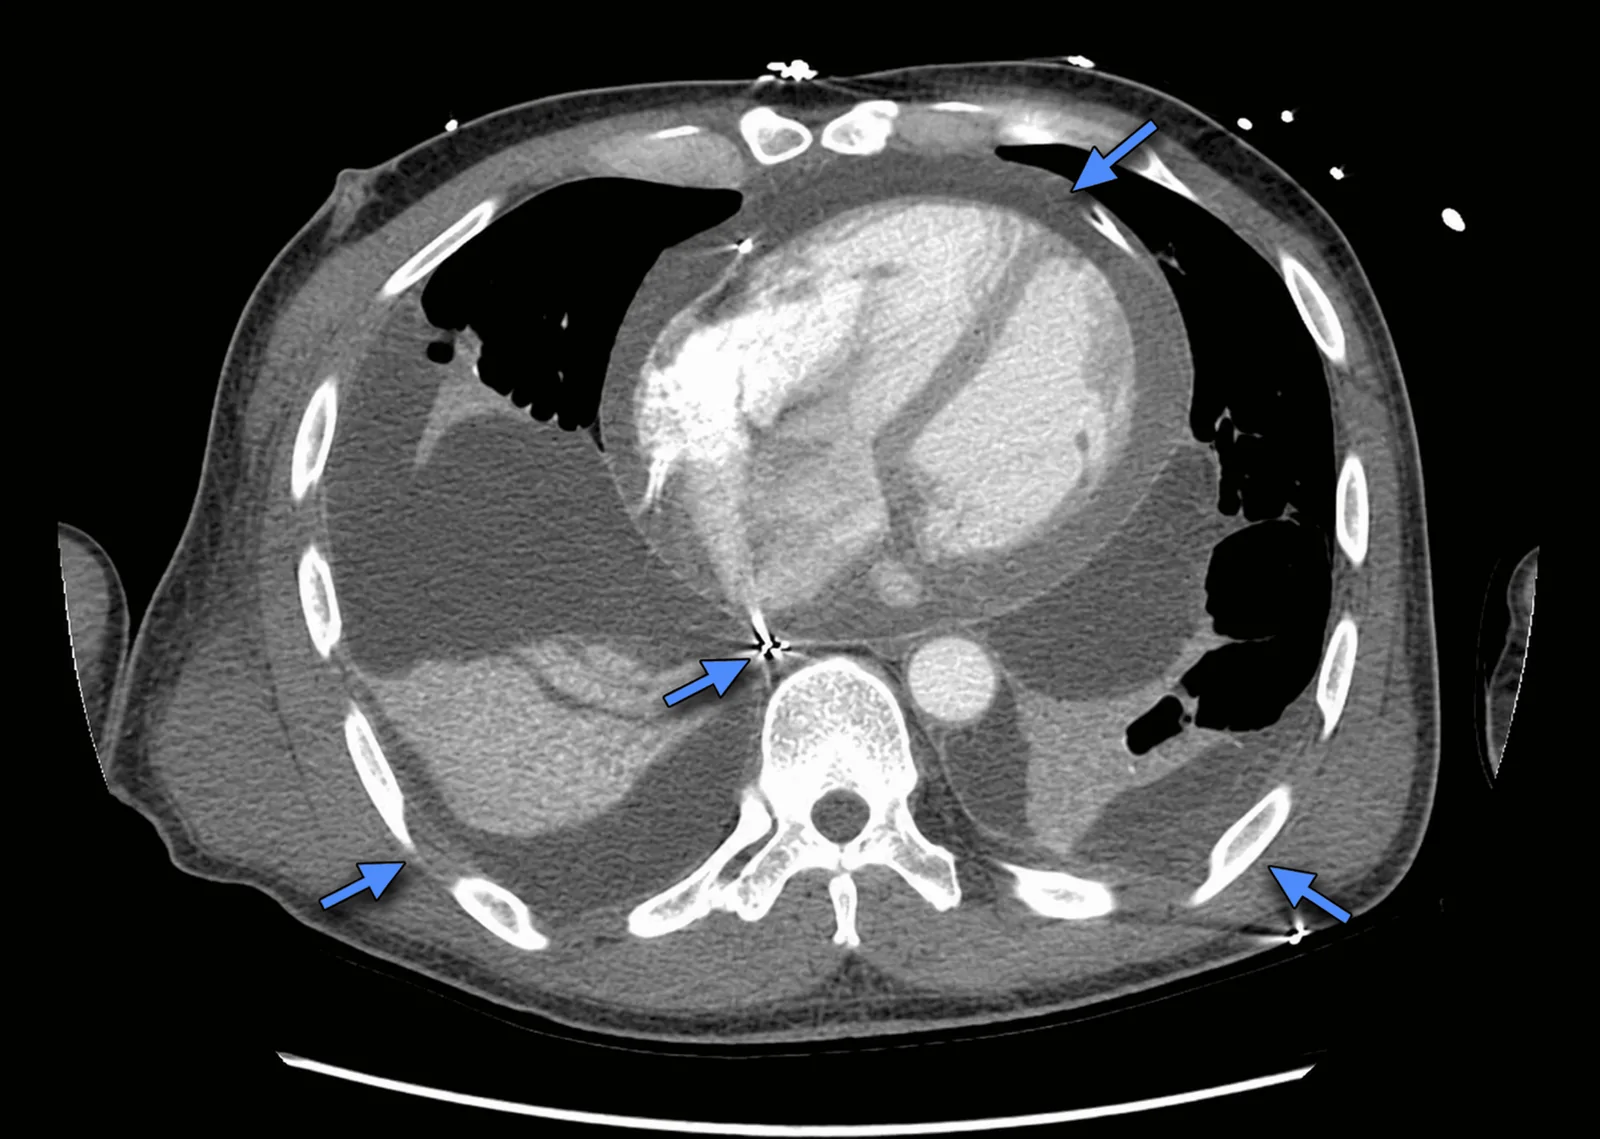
Computed tomography angiography (CTA) of the chest obtained after pericardiocentesis. The upper arrow indicates a residual moderate pericardial effusion. The central arrow indicates the pericardial drain in situ. The lower right and lower left arrows indicate persistent bilateral pleural effusions, including a moderate right pleural effusion and a small left pleural effusion, with associated partial collapse of the right and left lower lobes. Despite interval drainage, residual pericardial and pleural fluid collections remained. Underlying malignancy could not be excluded.
